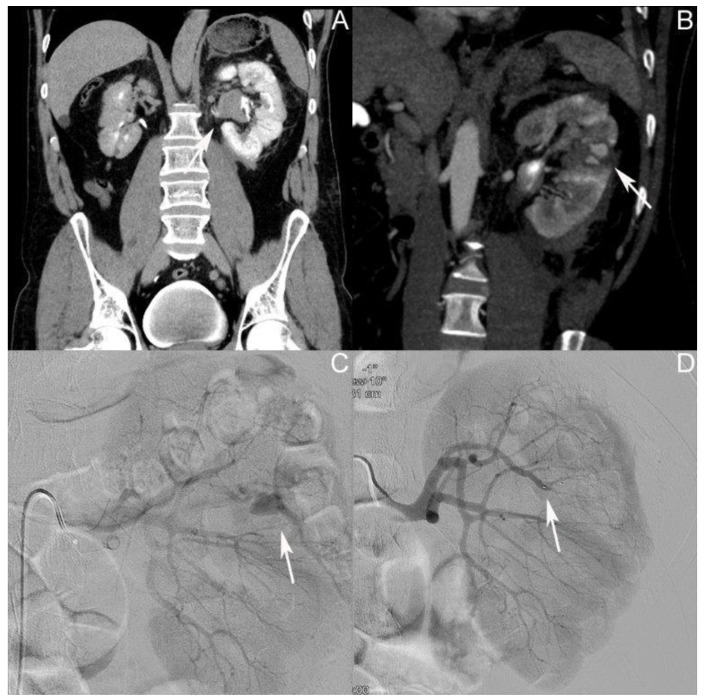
Selected imaging of case II. (A) Coronary contrast-enhanced computed tomography (CT) scan of the abdomen; the arrow shows the affected left kidney with coagula in the renal pelvis. (B) Coronary contrast-enhanced CT scan of the abdomen; the arrow shows the 15 mm arteriovenous (AV) fistula in the middle pole of the left kidney. (C) Angiography of the left kidney; the arrow shows the AV fistula. (D) Angiography post coil embolization; the arrow shows the coils in situ.
